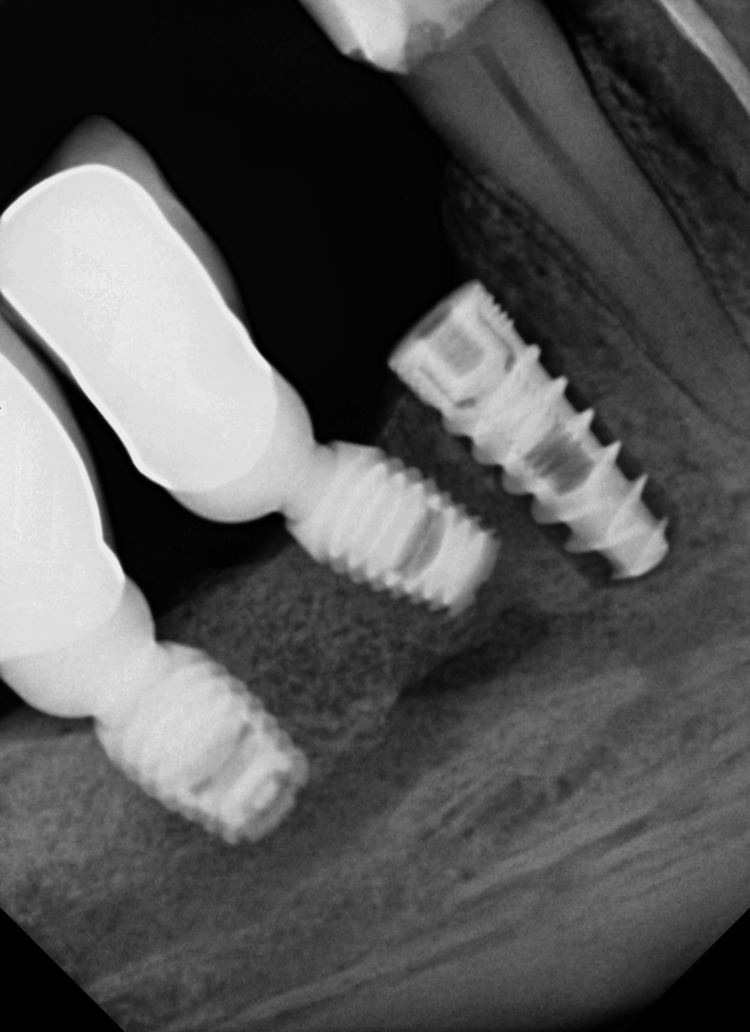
Control X-ray postinsertion implant (D: 3.75 mm, L: 10 mm)
